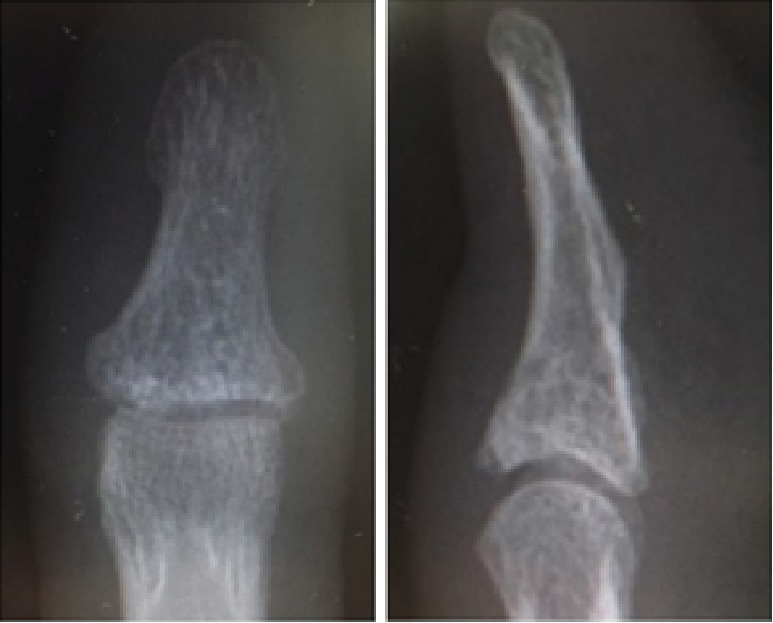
Follow-up radiographs showing the healed fracture.
